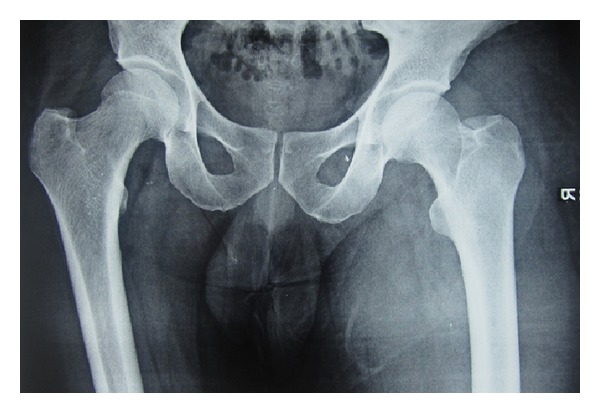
X-ray bilateral hip joint—X-ray showing a normal hip joint and femur with soft tissue swelling on right thigh.
